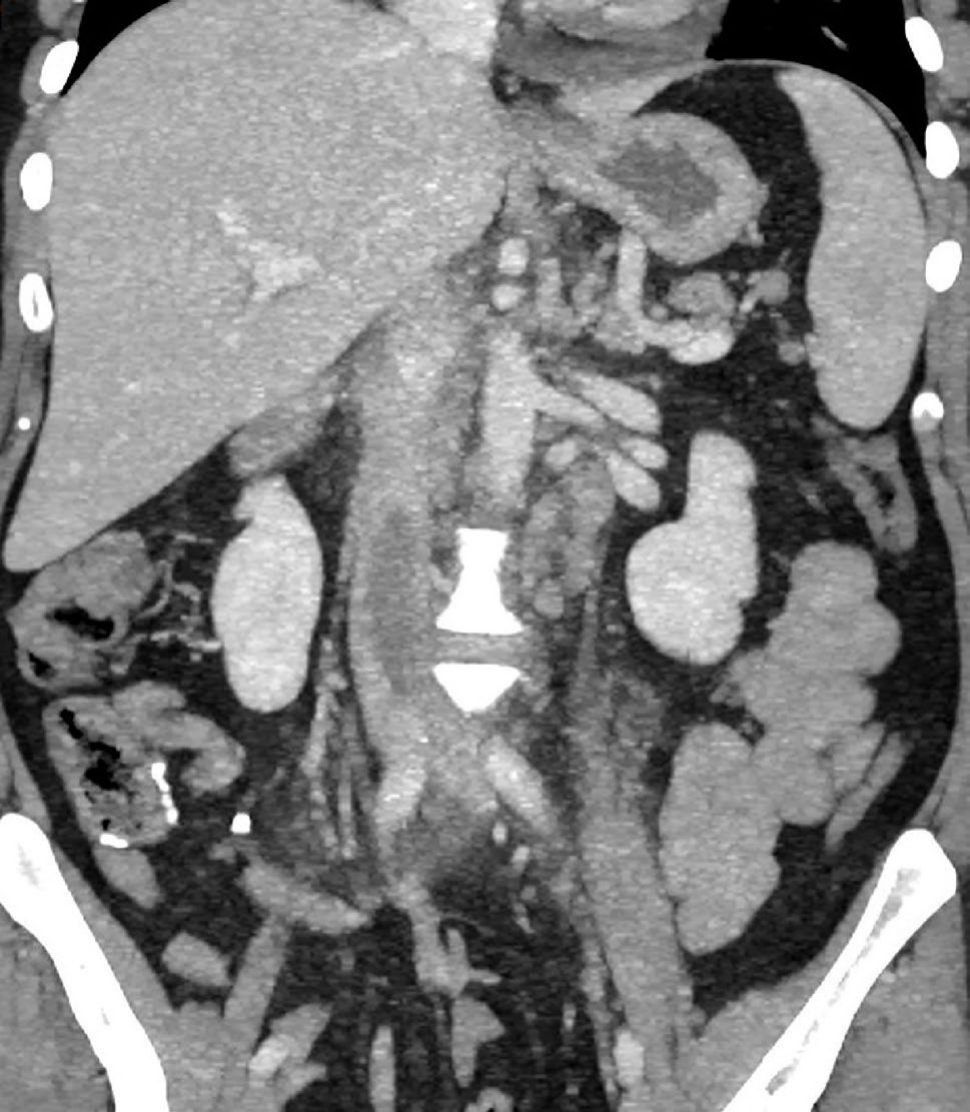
Coronal contrast-enhanced abdominopelvic CT image demonstrates an extensive thrombus within the inferior vena cava, extending into the left common iliac vein, accompanied by retroperitoneal lymphadenopathy and associated inflammatory changes
